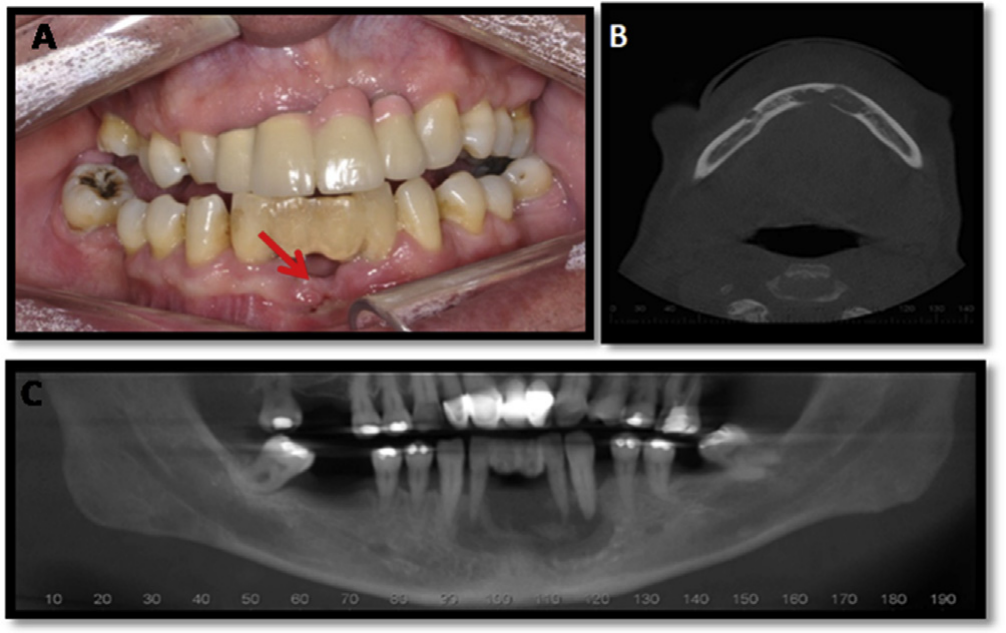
A. Intraoral image revealing initial clinical condition with drainage of purulent secretion. B. Axial computed tomography section revealing bucco-lingual extension of the lesion. C. Initial tomographic appearance of the lesion.
